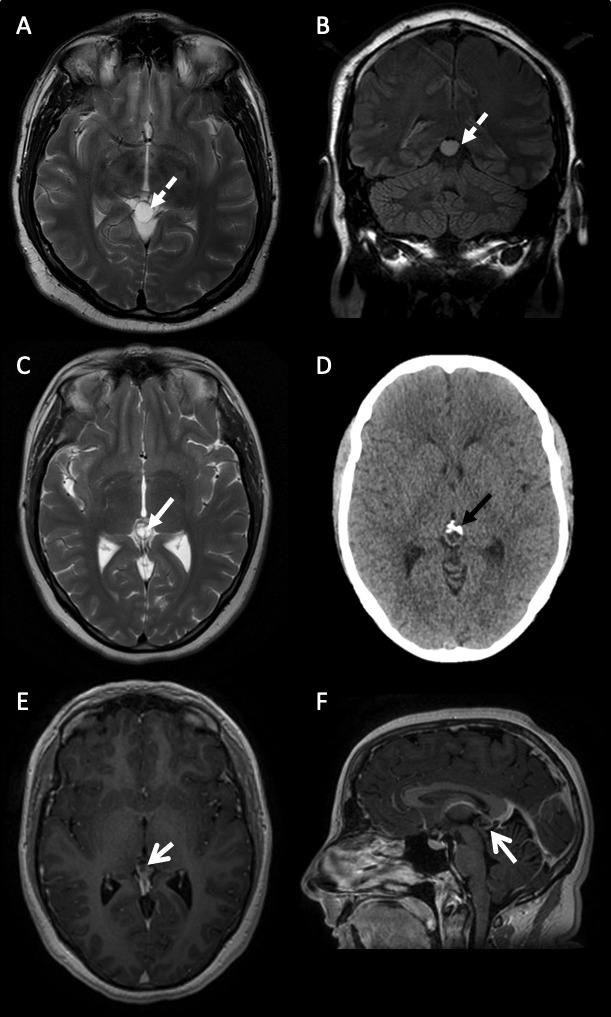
Varying MR brain scan features of pineal region cysts. (A) and (B) A 38-year-old man with a simple pineal region cyst (A) axial T2-weighted and (B) coronal FLAIR sequences of a simple unilocular pineal region cyst with no internal structure (dashed white arrow). The signal hyperintensity does not suppress on FLAIR imaging. (C–F) A 31-year-old woman with an atypical pineal region cyst. (C) Axial T2-weighted sequence of an atypical multilocular, septated (white closed arrow) pineal region cyst. (D) Axial CT scan of head showing anterior calcification (black closed arrow). (E) Axial and (F) sagittal gadolinium-enhanced postcontrast T1 sequences of an atypical septated, posteriorly enhancing (open white arrows) pineal region cyst. FLAIR (Fluid Attenuated Inversion Recovery).
